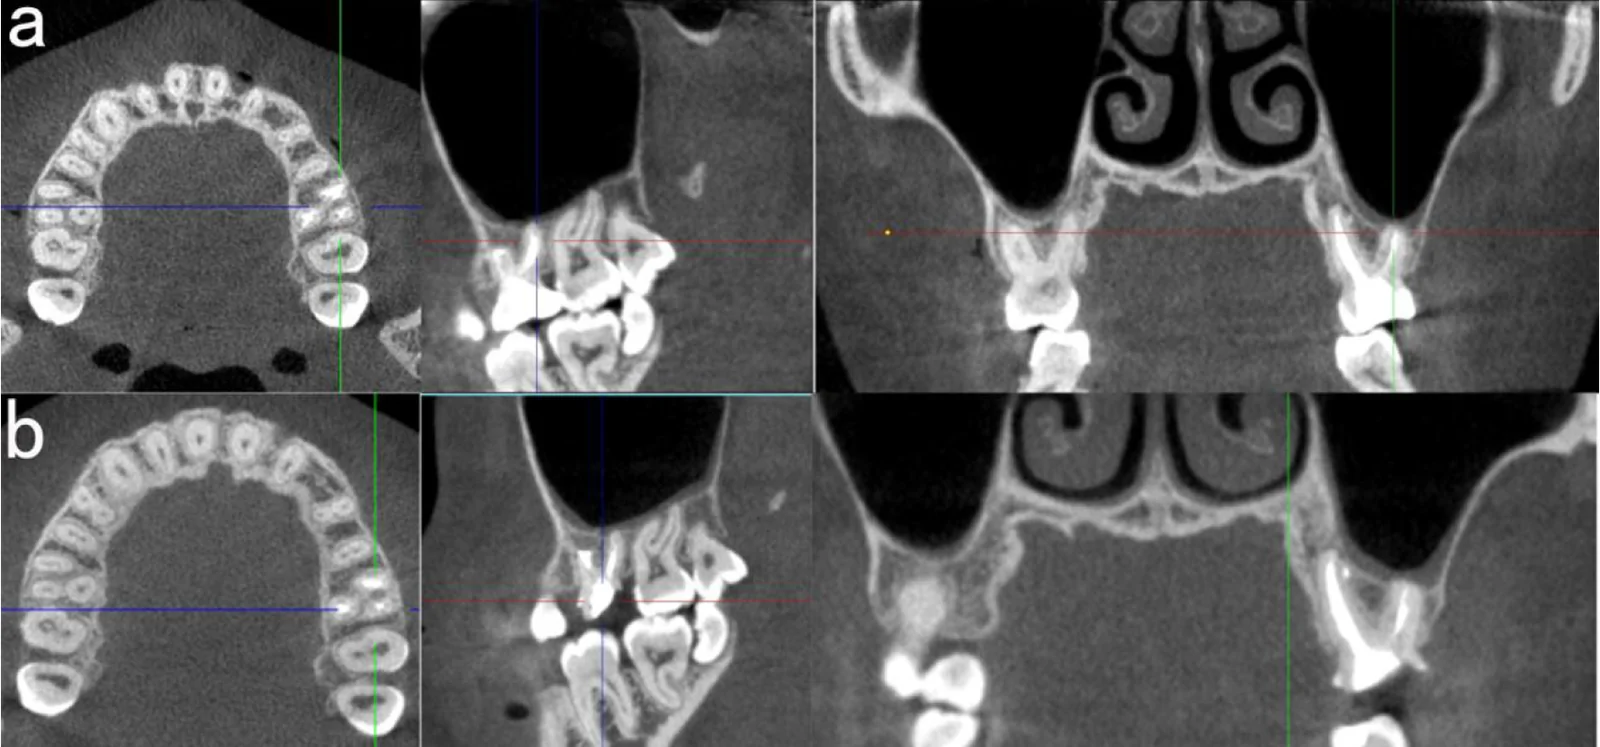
Postoperative CBCT. (a) 1-year follow-up; (b) 2-year follow-up. CBCT: cone-beam computed tomography.
